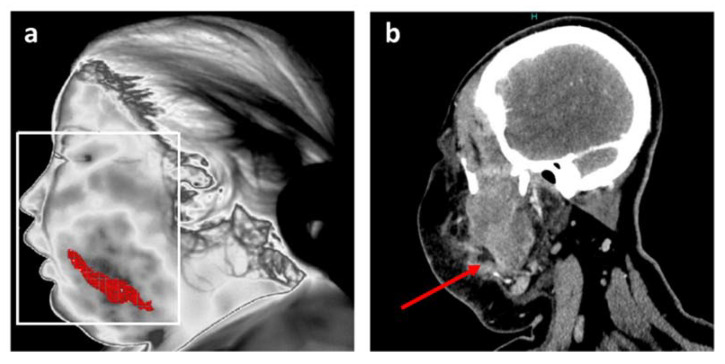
Position of the main inflammation site after thermal analysis for patient 1. (a) Resulting accurate localization of main inflammation linked to facial cellulitis with MIT; (b) Coronal plane of CT scan in soft tissue window at the level of the infectious collection (red arrow).
